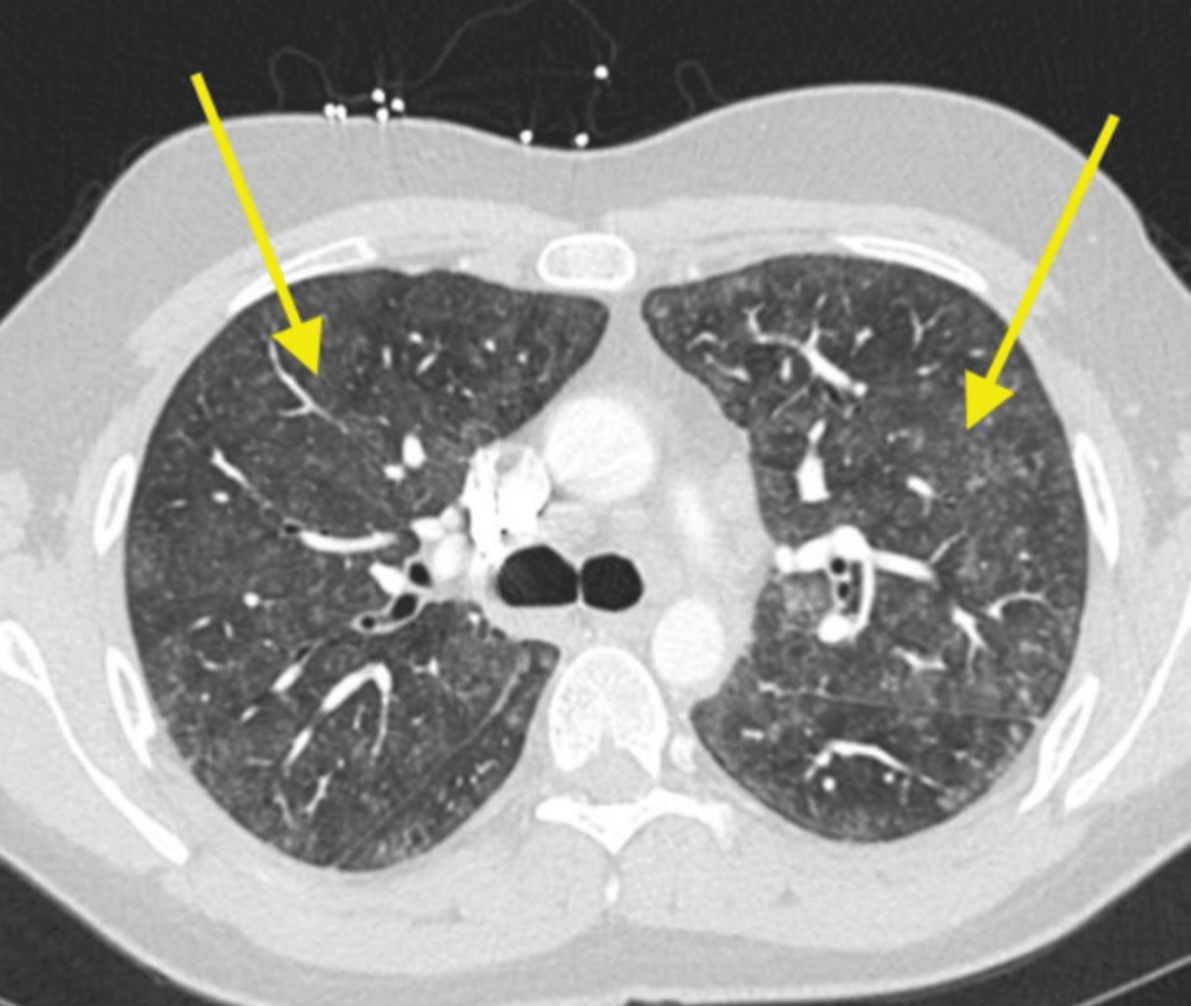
Chest CT showing diffuse centrilobular ground glass nodularity and bibasilar reticulations (yellow arrows)
